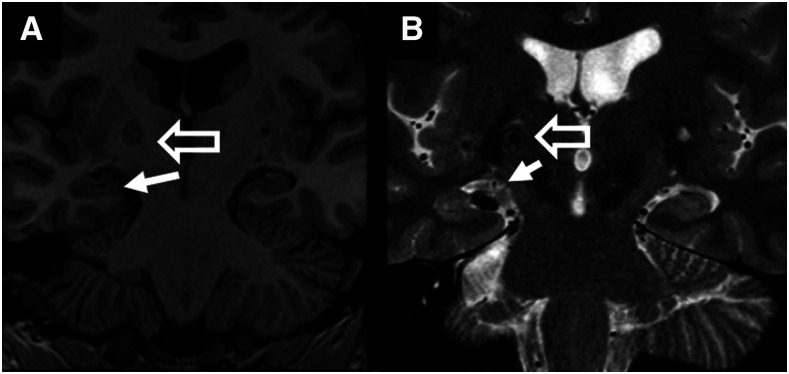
Hippocampal sclerosis. Hippocampal sclerosis (defined as the presence of hippocampal atrophy and a hyperintense signal on long-repetition-time sequences of the hippocampus) is seen on the R-side (solid arrow) of the T1 (A) and T2-FLAIR (B) coronal MRI scans. An adjacent calcified lesion (hollow arrow) is also shown.
